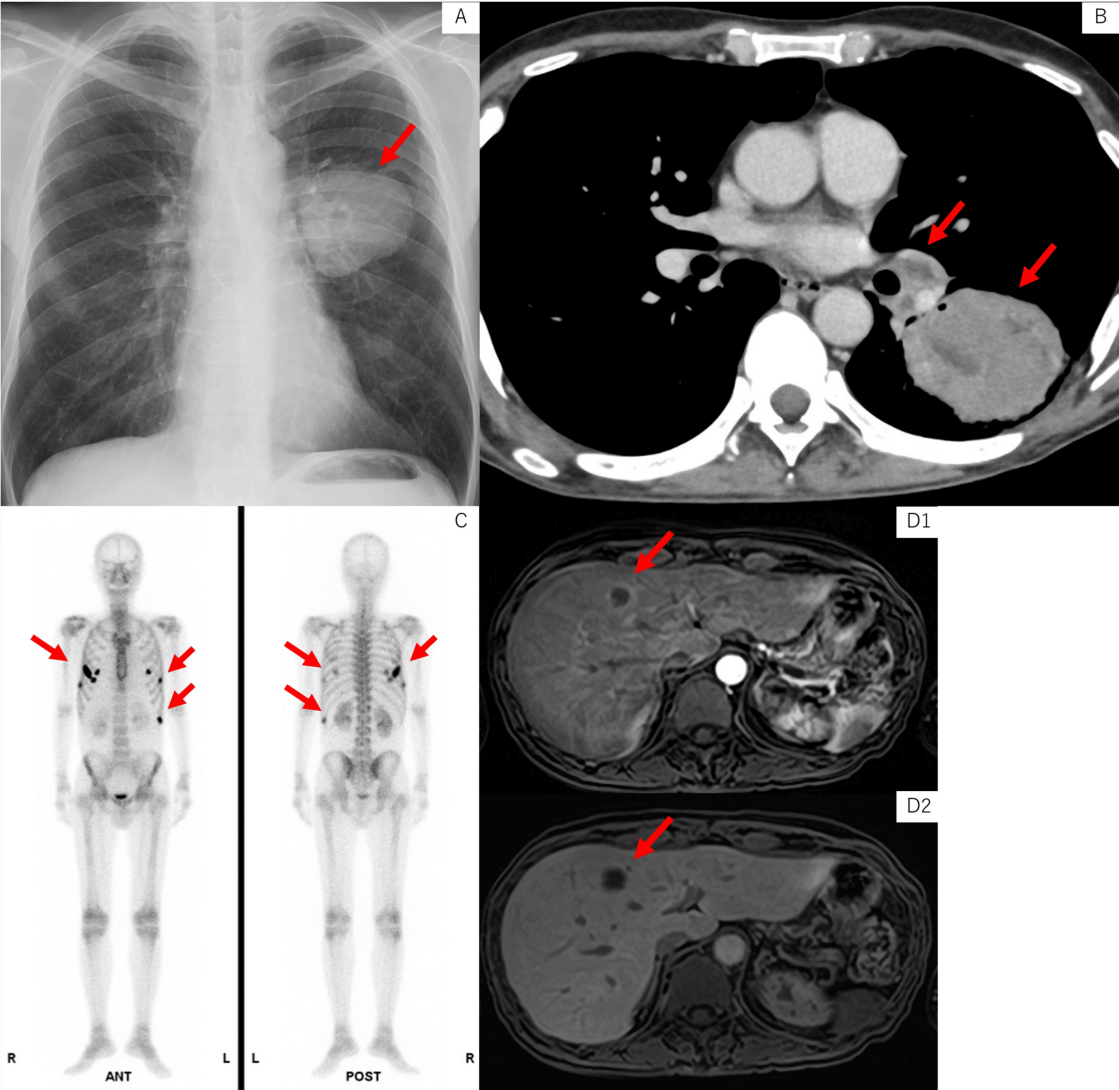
Imaging findings (A) Chest X-ray at the first visit showed a tumor shadow in the left middle lung field. (B) Contrast-enhanced computed tomography revealed a 73-mm oval mass in the left S6 and left hilar lymphadenopathy. (C) Bone scintigraphy demonstrated abnormal uptake in the right 4th, 5th, and 7th ribs and the left 6th, 9th, and 10th ribs. (D) Gd-EOB-DTPA-enhanced MRI of the liver demonstrated poor enhancement of the tumor interior, ring enhancement, and decreased EOB uptake. (D1: arterial phase; D2: hepatobiliary phase) Gd-EOB-DTPA, gadolinium ethoxybenzyl diethylenetriamine pentaacetic acid; MRI, magnetic resonance imaging
